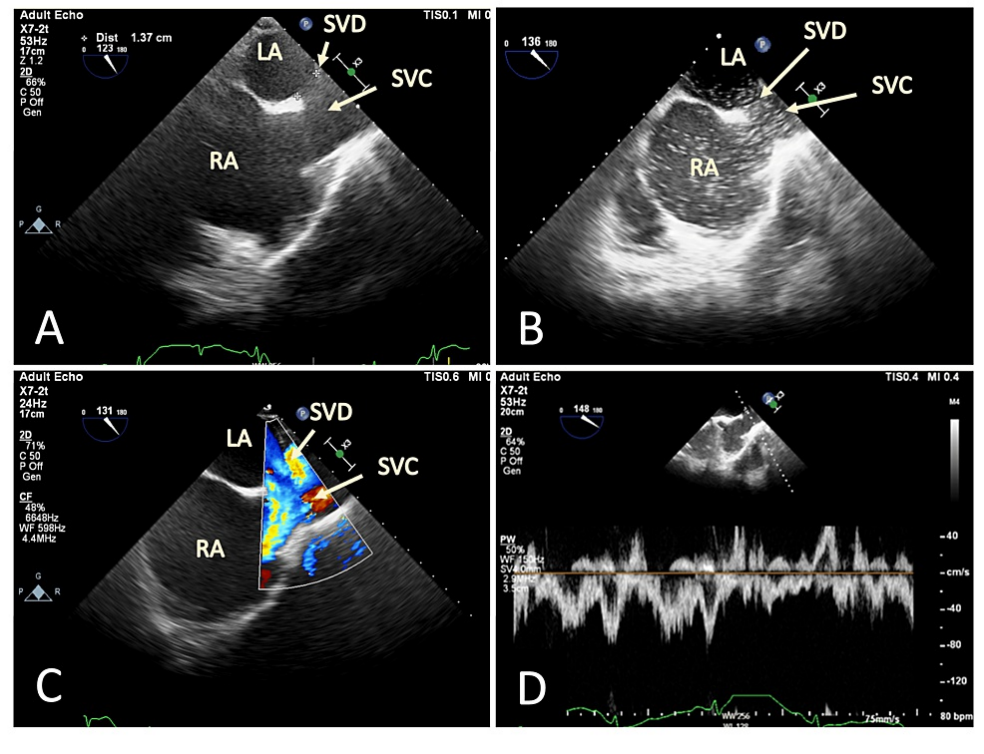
TEE evaluation of right-to-left shunt demonstrating the SVD with high flow concerning for an APVR (A) TEE of modified bicaval view demonstrating the large SVD. (B) With a marked amount of right-to-left shunt of agitated saline injected through an upper extremity peripheral vein. (C) Color flow doppler. (D) Pulse wave doppler (C and D demonstrate a bidirectional shunting and suggesting a predominant flow from the RUPV into the SVC). TEE: transesophageal echocardiogram; RV: right ventricle; RA: right atrium; LV: left ventricle; LA: left atrium; SVD: sinus venosus defect; SVC: superior vena cava; APVR: anomalous pulmonary venous return; RUPV: right upper pulmonary vein.
